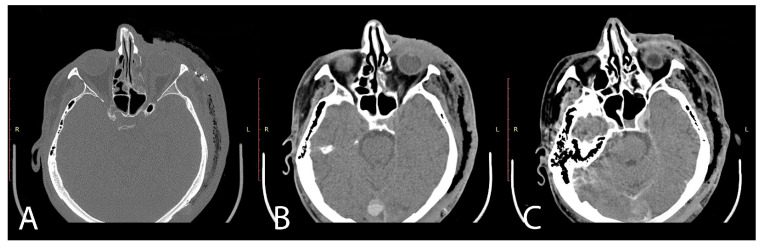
MRI examination: (A–C) subcutaneous emphysema and edema of the left hemiface.
